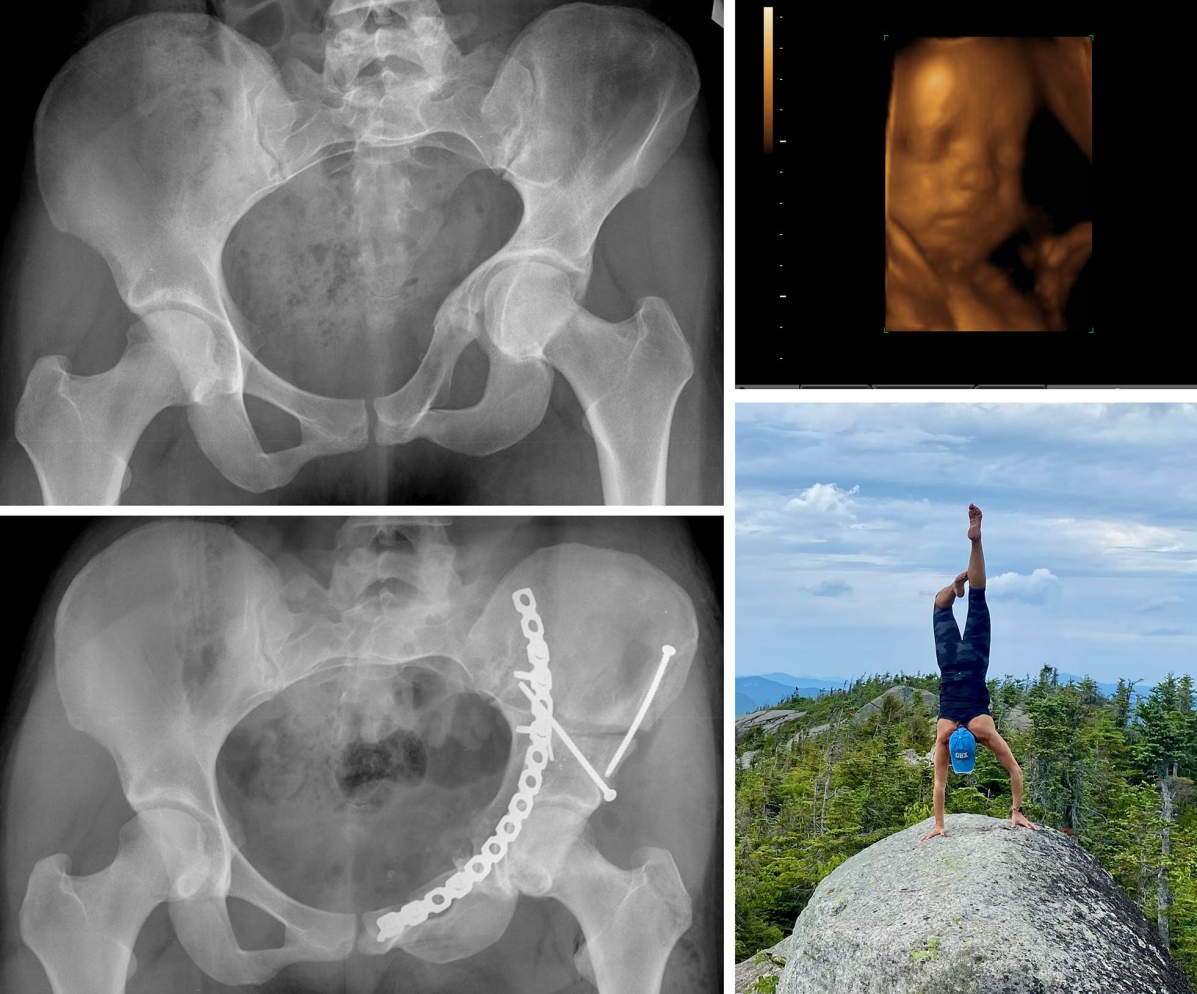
Posttraumatic protrusio observed in patient. a Posttraumatic protrusio and deformation of the hemipelvis in a 23-year-old female. Her gynecologist discussed the risk of abortion due to deviation of the uterus, which was the reason why she was asking for correction. A modified PAO was first tested on a plastic model. Correction of protrusio und pelvic deformation was assisted by a distractor. b The new position was stabilized with a long recoplate placed along the pelvic brim. Ultrasound was performed just before natural delivery. c At 16 years after surgery, she is now the mother of five children and is very active in outdoor activities. The straight leg is the operated one
